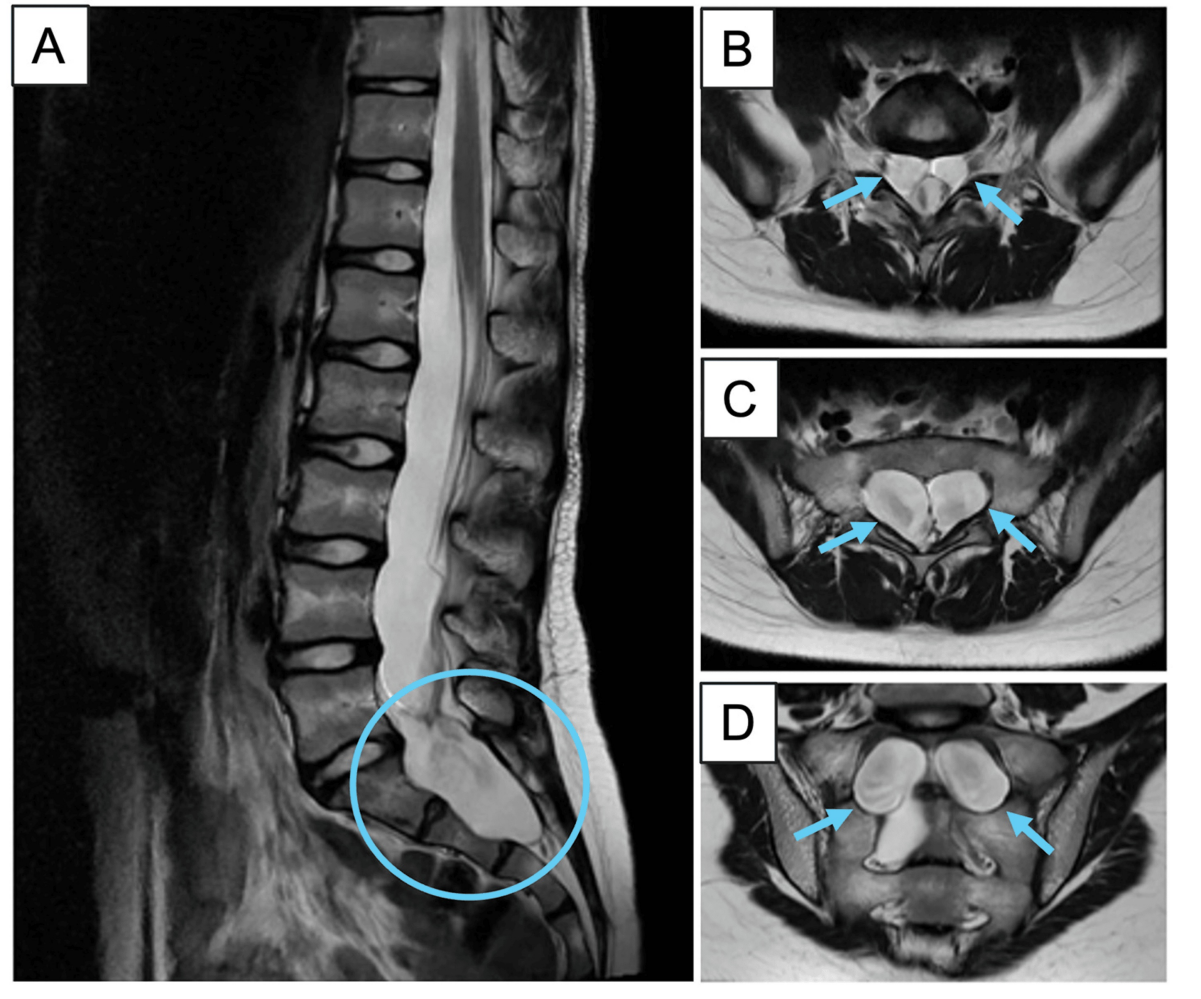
Representative T2-weighted MRI lumbosacral spine images in sagittal (A) and axial (B, C, D) directions. There are prominent perineural cysts (dimensions: right 5 × 5  × 2.3 cm and left 4 × 4 × 2 cm) surrounding bilateral S1 and right S2 cauda equina nerve roots (highlighted in light blue). MRI: magnetic resonance imaging
